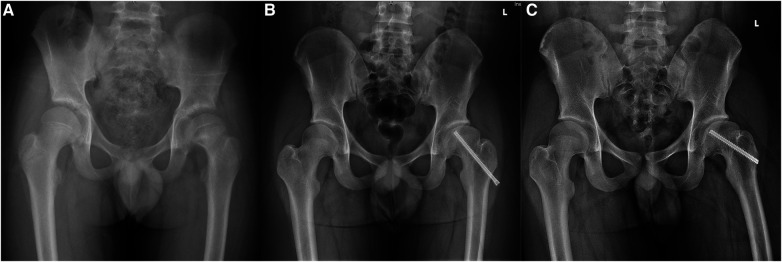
Radiographs of a 17-years old boy with difficult screw removal on the left side. (A) Pelvic radiograph of a 10-year-old boy with left slipped capital femoral epiphysis. (B) Preoperative pelvic radiograph of the patient at the age of 17 years old when planned to remove the screw. (C) Removal of the screw through an open approach was difficult and unsuccessful. The screw was not removed entirely.
